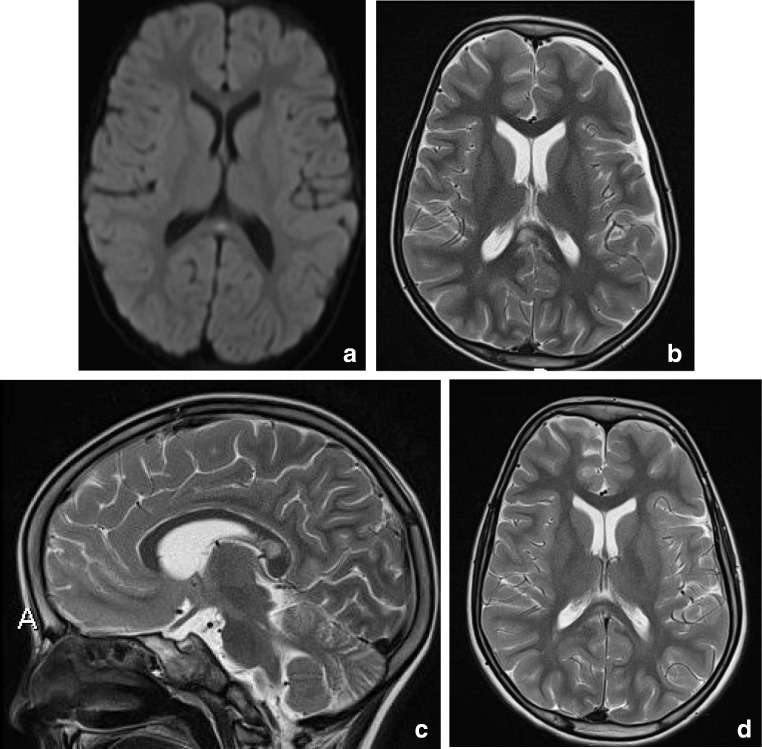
(a) Three-year-old girl with gastroenteritis by enterovirus. Axial DWI (a) on day 4 shows a small lesion with unsharp border consistent with a RESLES lesion. (b-d) Six-year-old girl with a pneumococcal meningitis following an ear infection. Axial and sagittal T2 at admission (b, c) shows an area of edema in the splenial and dorsal isthmus (no DWI was made). At 5-year follow-up, a small residual lesion is seen on the axial T2 (d)
